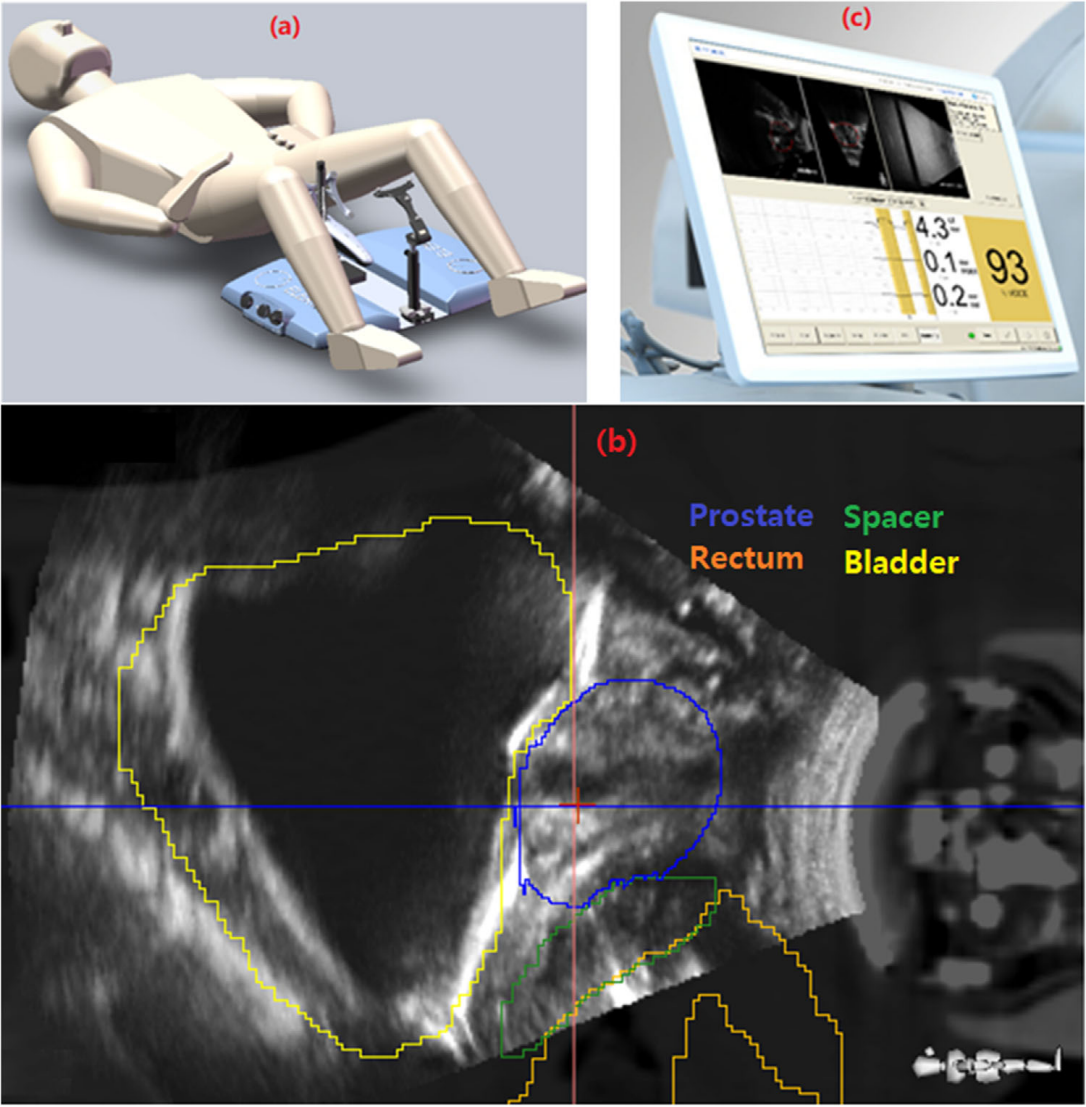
Workflow of ultrasound guided prostate SBRT. (a) Patient setup with the base plate and the ultrasound probe, (b) CT/ultrasound registration with structure contours, (c) intra‐fraction motion monitoring using Clarity Autoscan.
